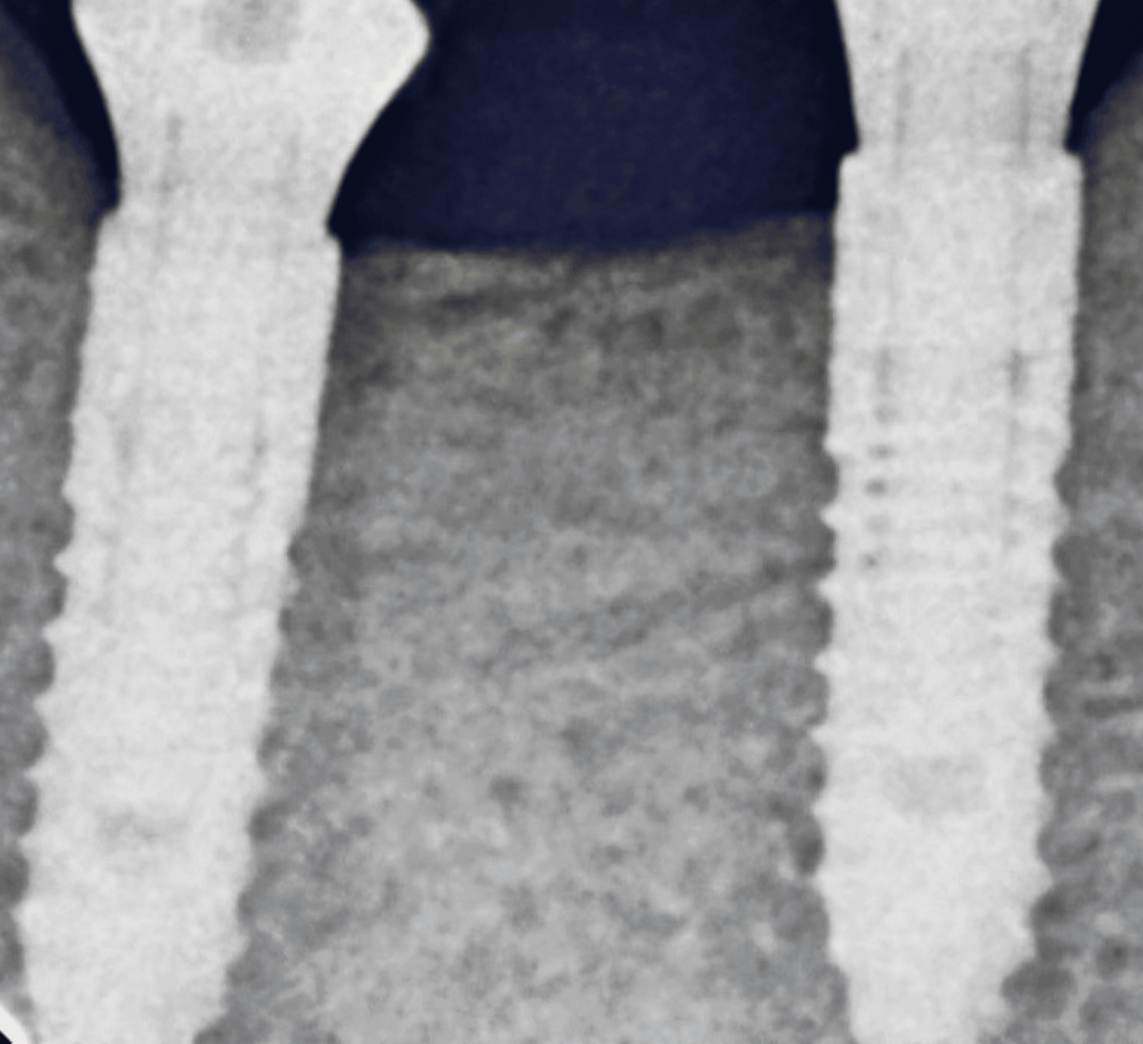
Postoperative radiograph after three months
